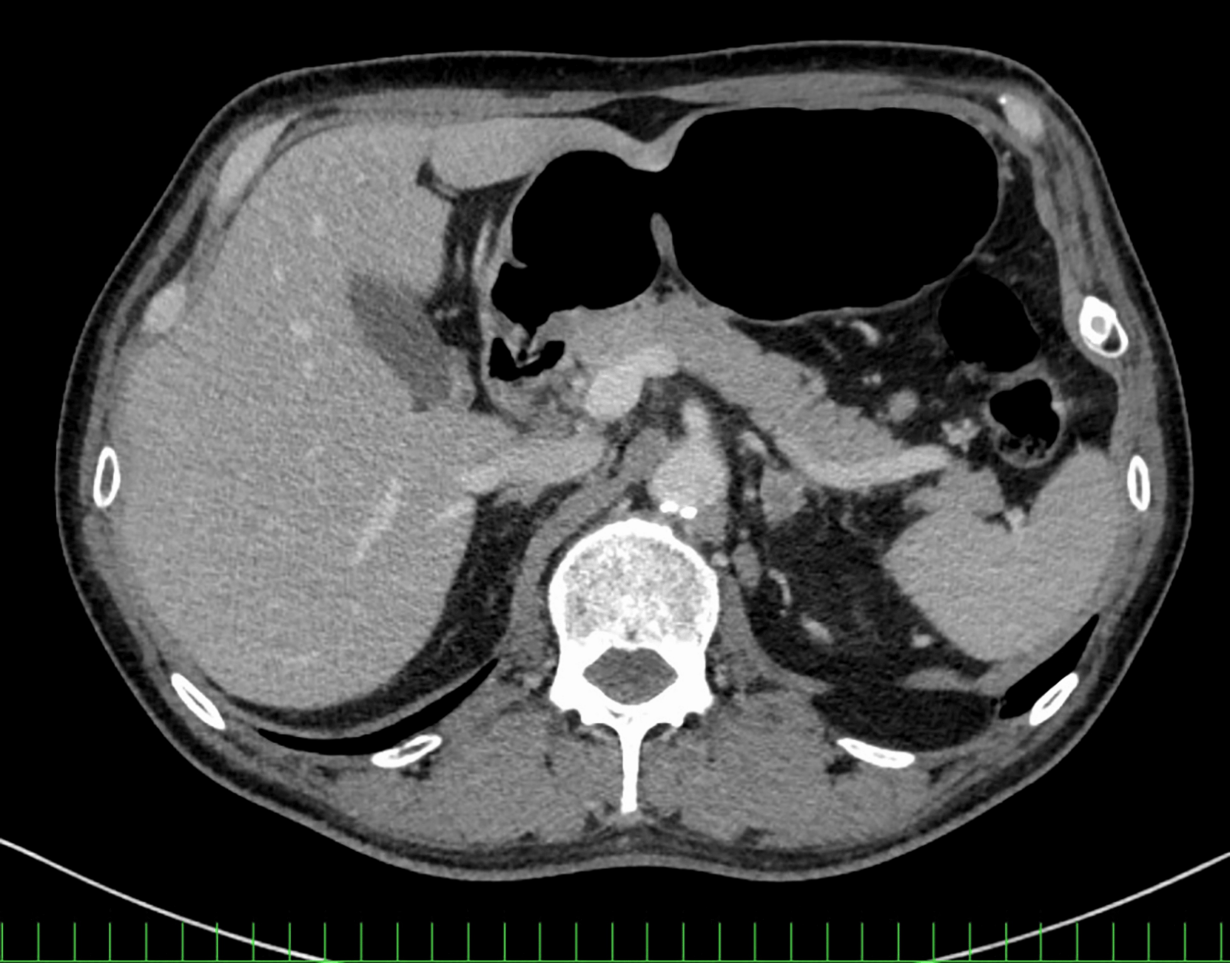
Follow-up imaging of Case 2 after selpercatinib therapy. Contrast-enhanced CT showing no visible liver metastases compared with baseline imaging.
